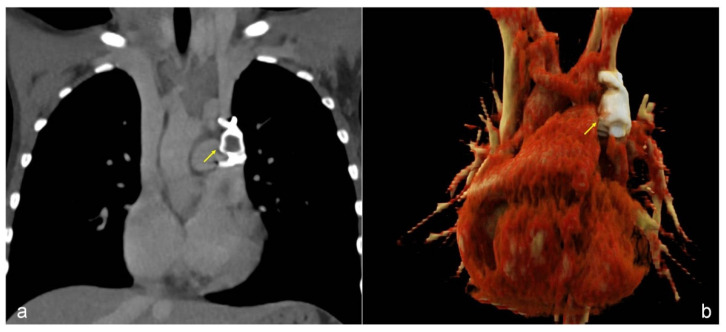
(a). Computed tomography two months after occlusive device insertion (arrow). (b). A 3D-volume rendering image shows proper position of the plug and confirms left SVC occlusion. Blood reaches the right SVC through the bridging vein; its current diameter is 9 mm.
